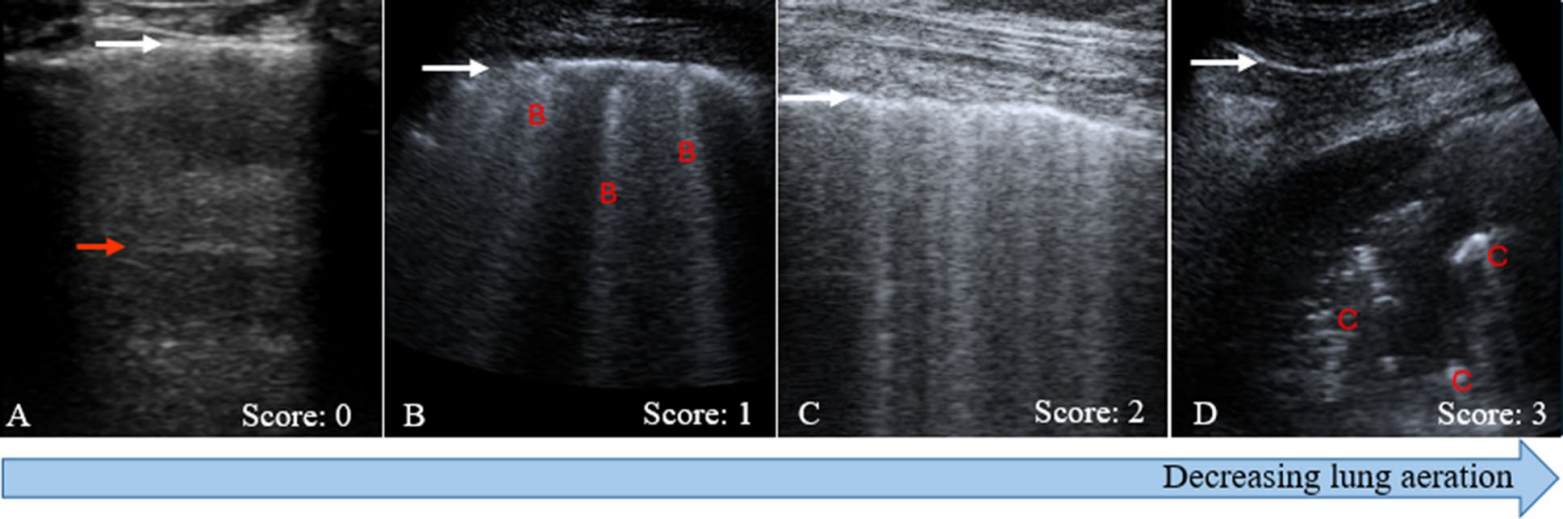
Principles in lung ultrasound (LUS) and profiles. A Normally aerated lung (LUS aeration score 0) showing the pleural line (white arrow) and A-line (red arrow) which represents a reverberation artefact of the pleural line. B Mild aeration loss (LUS aeration score 1) with three B-lines denoted in red. C Moderate aeration loss (LUS aeration score 2) where the B-lines take up more than half of the pleural line. D Severe aeration loss (LUS aeration score 3) with consolidations indicated in red
